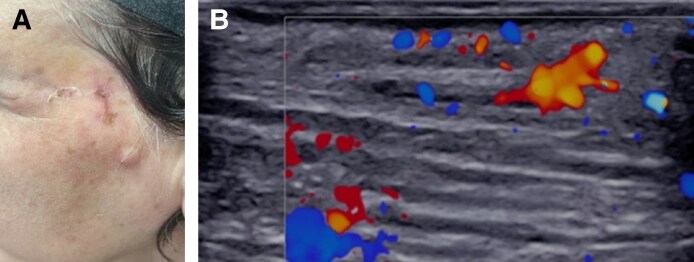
Clinical and ultrasound evaluation 4 days after initial hyaluronidase treatment (Case 3, a 60-year-old woman with diabetes). (A) Resolution of the patient's clinical symptoms. (B) Transverse color Doppler ultrasound showing partial flow restoration in superficial temporal artery. (C) Clinical image of patient 2 days after injection of additional 300 units hyaluronidase.
